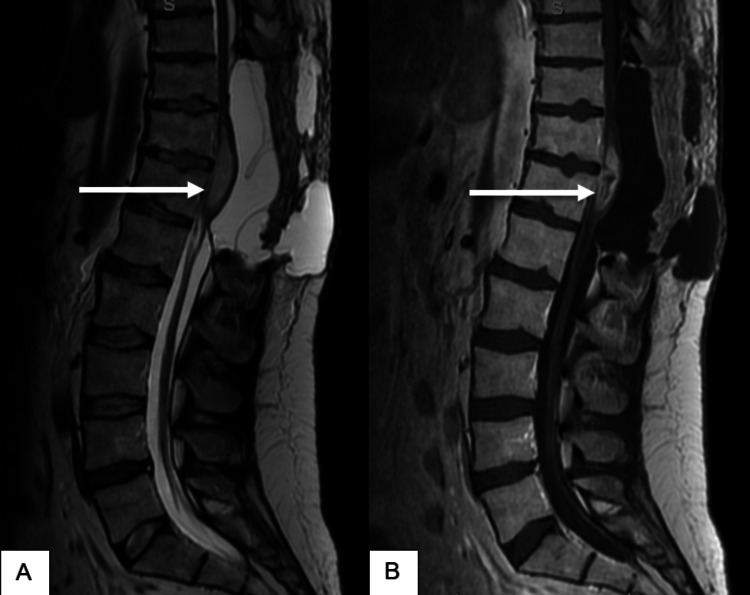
MRI of the lumbar spine post-subtotal removal of tumor, sagittal view (A) T2-weighted and (B) T1-weighted post-contrast images showing residual enhancing intramedullary mass (white arrows) at the T11-12 level.
